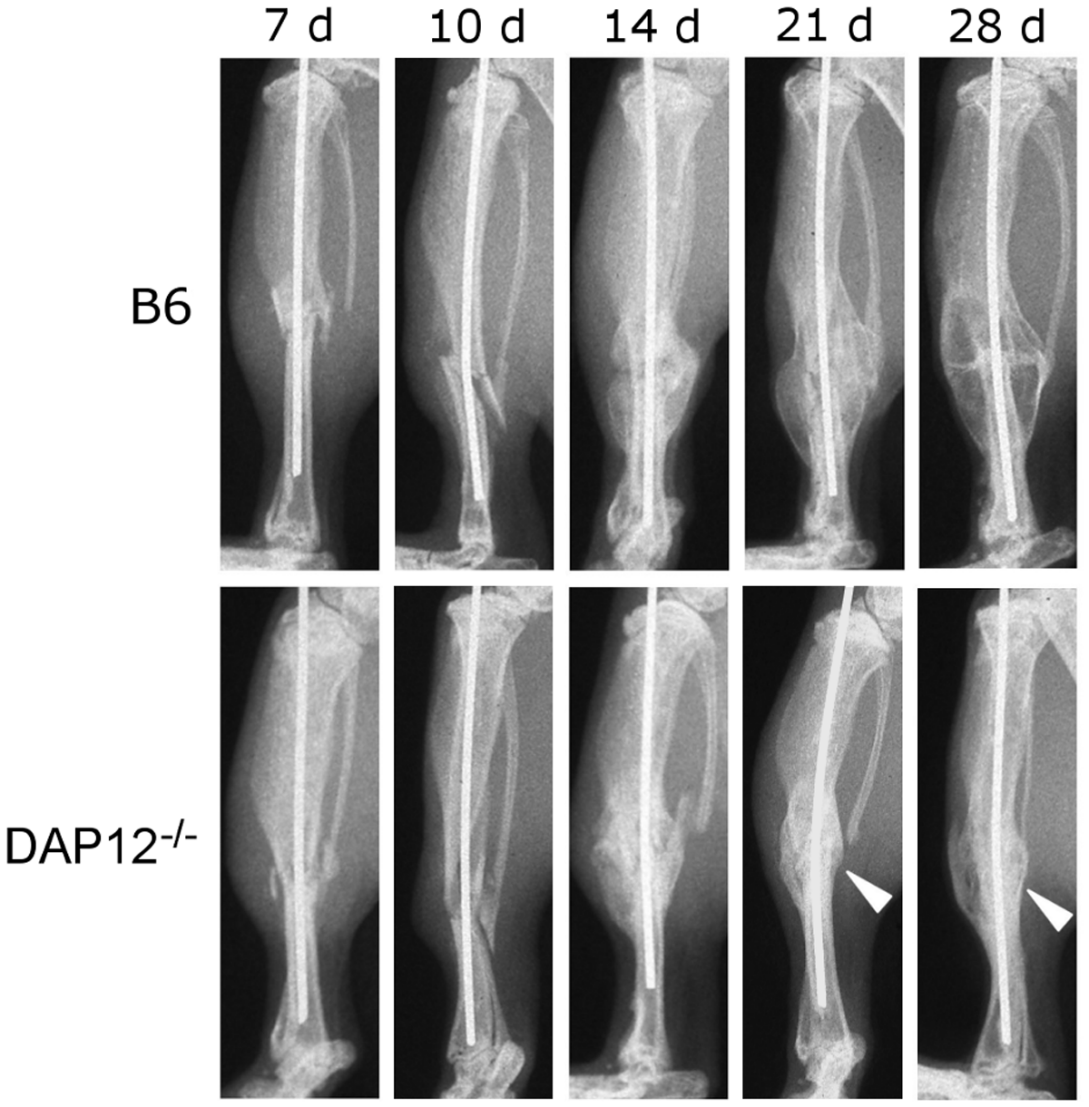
Radiographic images of fractured tibiae in B6 and *DAP12*
